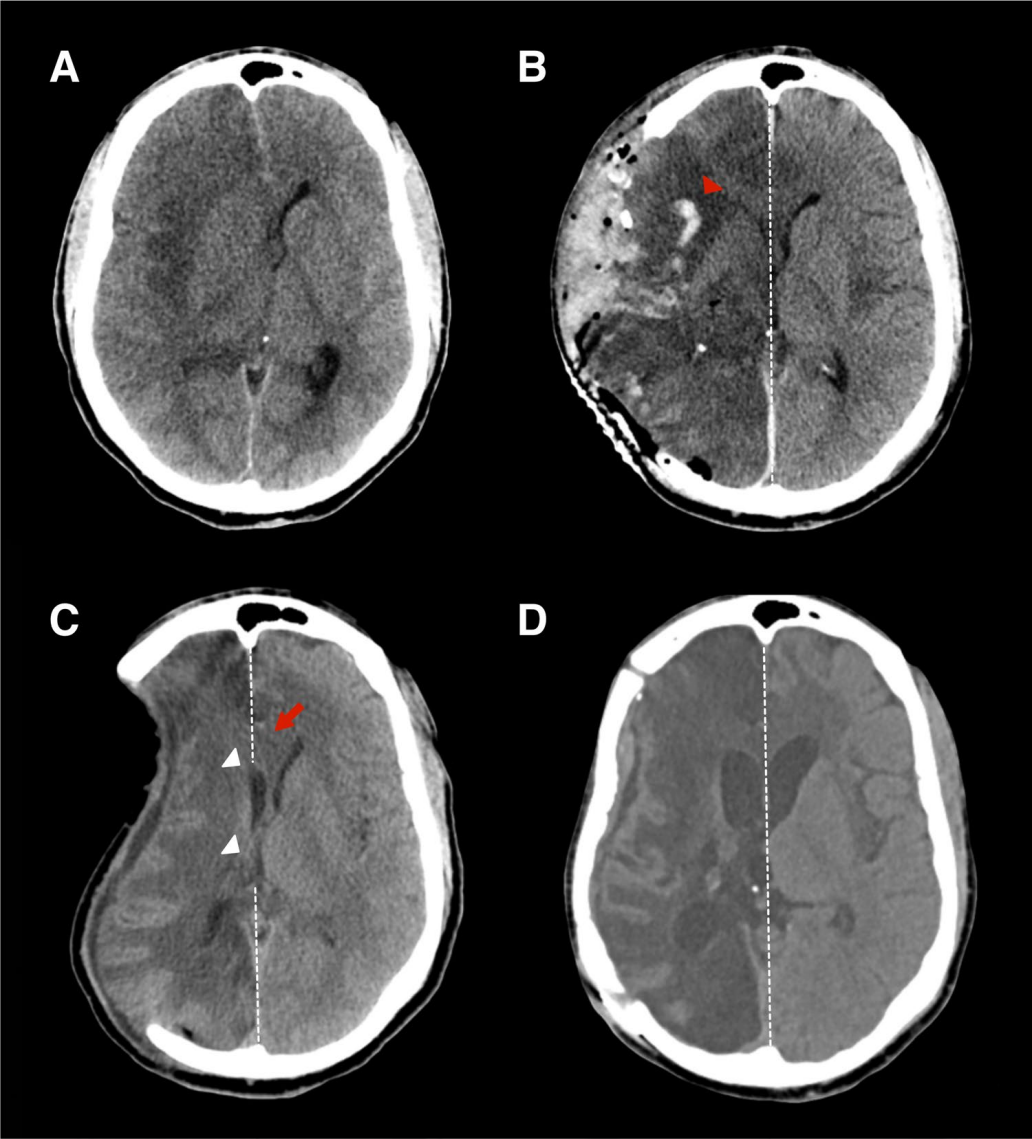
Serial imaging of SoT. *Legend*: A A right-handed individual without previous medical history underwent DC and right temporal lobectomy due to HSV-1 encephalitis induced brain edema. B The postoperative CT showed decompression of swollen brain tissue and a new intraparenchymal hemorrhage (red arrowhead). C Ten weeks after DC, the patient developed nausea and vomiting, worsening left-sided paresis, aphasia, decreased level of consciousness in a vertical position (GCS decrease from 11 to 8), and improvement to baseline in a supine position. Based on these clinical findings, an “a priori” SoT was diagnosed. CT imaging at 10 weeks showed the craniectomy site’s sinking appearance, paradoxical deviation of midline structures (red arrow), slit third ventricle, and AHLV (white arrowheads). D Cranioplasty was performed at week 11, resolving the radiologic signs of SoT and orthostatism. After cranioplasty, the motor function returned to baseline, consciousness improved (GCS 14), and the patient started to communicate in writing. Abbreviations: DC, decompressive craniectomy; HSV-1, herpes simplex virus type 1; GCS, Glasgow Coma Scale; AHLV, anterior horn of lateral ventricle; SoT, syndrome of the trephined
